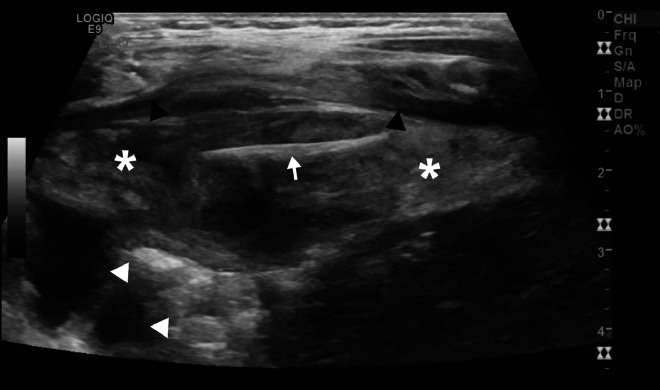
Abdominal ultrasonographic image at the left lower quadrant shows a well-defined fusiform-shaped, heterogeneous, predominantly hypoechoic mass (asterisks) resembling a localized fluid collection, measuring 7.0 × 2.5 cm (length x depth), is observed adjacent to the anterior abdominal wall (black arrowheads) and the colon (white arrowheads). Within this collection, there is a linear echogenic structure (arrow) measuring approximately 2.3 cm in maximum length, suggestive of a suspected foreign body.
